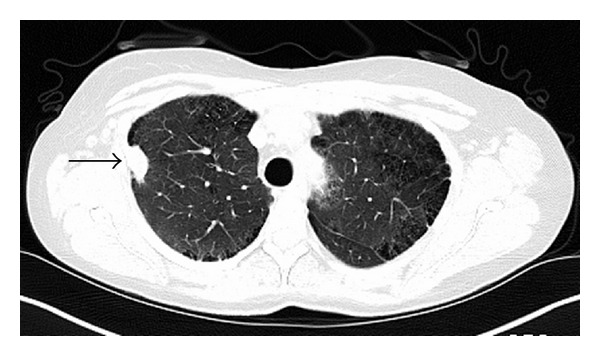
CT chest showing 1.5 cm × 2.5 cm RUL pleural-based nodule (arrow).
